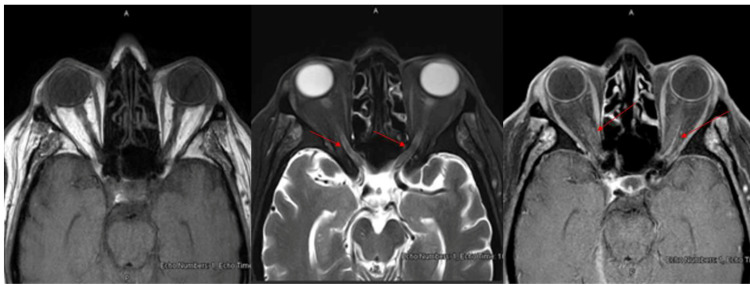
MRI orbits with gadolinium axial view (T1WI, T2WI, T1WI-Gad.) MRI orbits with gadolinium with axial view showed distal intra-orbital optic nerve sheath faint enhancement without extension to proximal intra-orbital or pre-chiasmatic optic nerve segment, normal appearance of the uveoscleral coat and extraocular muscles (as shown by the red arrows). T1-weighted image (T1WI), T2-weighted image (T2WI), T1WI with gadolinium (T1W1Gad.)
